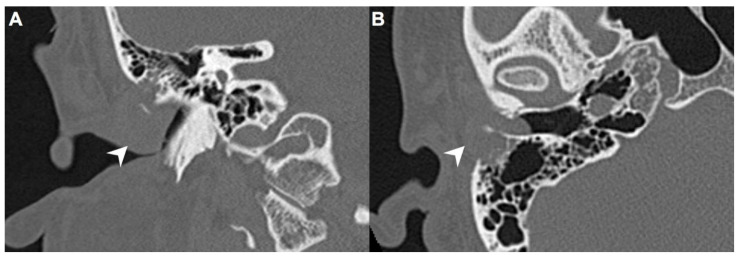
(A) On high-resolution computed tomography (HRCT), right EAC was occupied by a mass (arrow) on coronal view. (B) This submucosal soft tissue mass (arrow) was accompanied by partial mastoid bony destruction on axial view.
